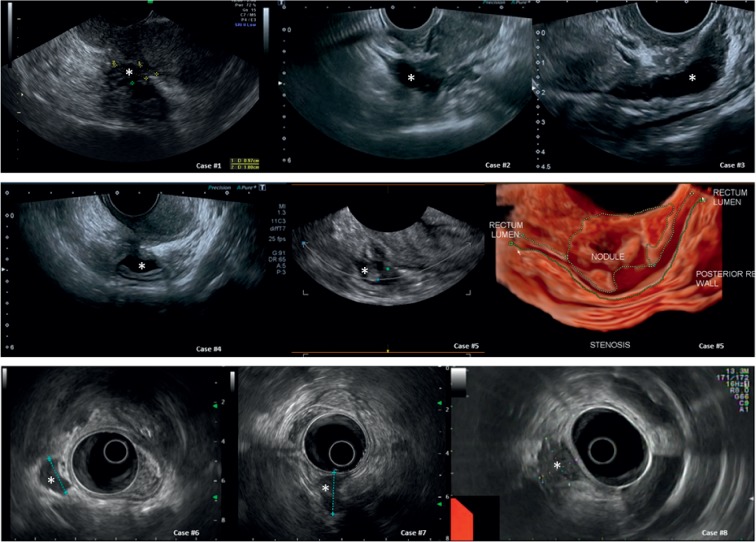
Images of bowel endometriosis (sonographic details of each case are reported in Table 1).
